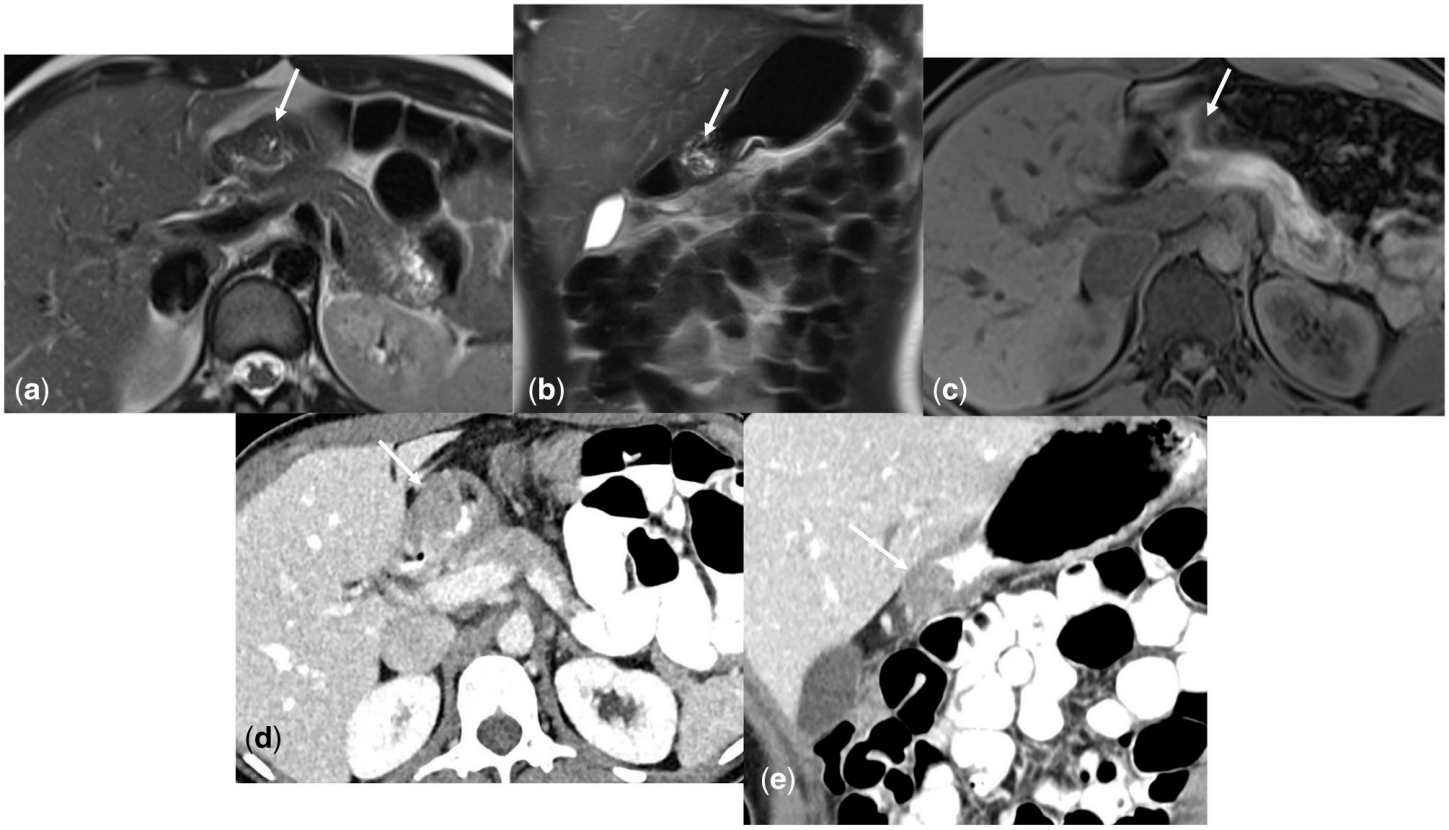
False Positive Finding on Screening WB-MRI. 38-year-old asymptomatic patient underwent screening whole-body MRI (WB-MRI) without IV contrast, including axial and coronal T2-weighted single-shot fast spin-echo (SSFSE) sequences (a, b) and T1-weighted gradient-recalled echo (GRE) sequence (c), which demonstrated a 1.4 cm T2 isointense lesion in the gastric antrum with internal foci of T2-hyperintensity (arrows a and b). The lesion was poorly visualized on the T1-weighted sequence (arrow, c) due to artifact and was not visualized on diffusion weighted imaging (not shown). As this was poorly visualized on MRI due to artifact from adjacent bowel gas, a contrast enhanced abdomen CT was recommended, redemonstrating the antral mass on axial and coronal CT (arrows d and e). This was interpreted as a gastric mass, possibly a gastrointestinal stromal tumor. The patient underwent upper endoscopy and biopsy with results yielding ectopic pancreatic tissue.
